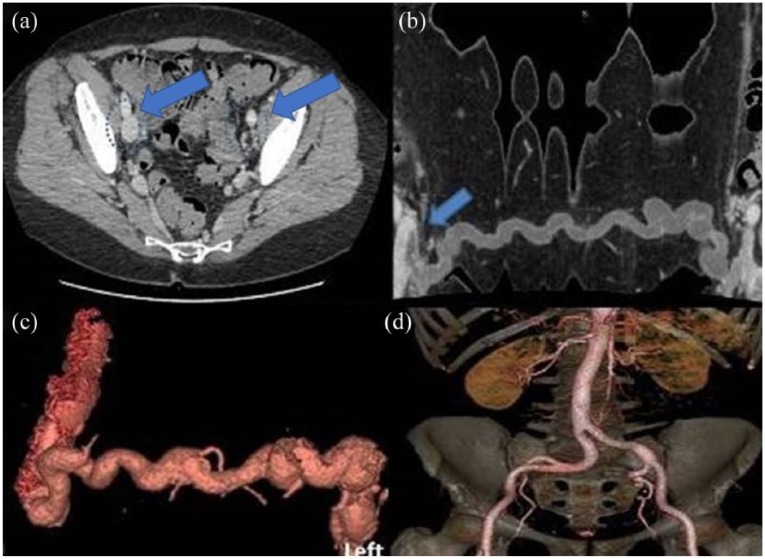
(a) Axial CT image showing the left common femoral vein appearing thread-like in size and unopacified due to suspected chronic thrombosis. Note the asymmetry with the average right size. (b) Curved reconstructed CT image showing the exact course of the collateral vein which is seen connecting the two common femoral veins. Note is made of the freely floating thrombus occupying the whole extent of the collateral and partially the common femoral vein lumen (arrow). (c) Three-dimensional (3D) volume rendering reconstructed CT image showing the collateral vein and the common femoral veins in three dimensions. (d) Virtual reconstruction of arterial phase.
